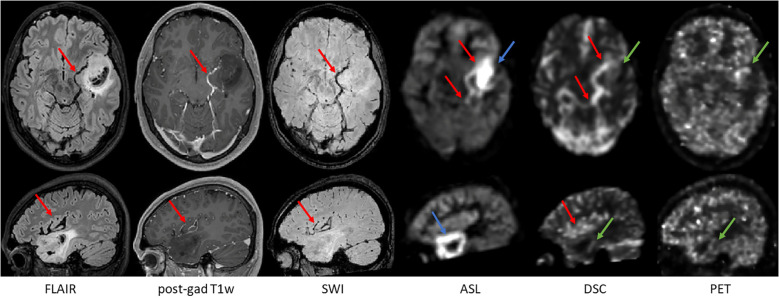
Participant 6 (grade 2 astrocytoma, IDH mutant, male, 20y) - a pair of representative axial (top row) and sagittal (bottom row) slices. On post-gadolinium T1w, the tumor shows inhomogeneous peripheral enhancement. On the axial images, a dilated draining vein is visible medial to the tumor on FLAIR, T1w, SWI, ASL, and DSC (red arrow). While the flow is likely high through this dilated vessel, the velocity is likely relatively low. This leads to a long arrival time, making the vessel visible on ASL and DSC (as indicated by the two red arrows), despite the absence of other macrovascular artifacts on ASL. On the sagittal images, a convolution of displaced and dilated M1 and M2 vessels running right above the tumor is visible on FLAIR, T1w, and SWI, with increased signal also on DSC, but not on ASL and PET (red arrow). Blood flow and volume comparable to that of normal GM were present in the tumor rim as assessed on PET and DSC (green arrows), and on the arterial blood volume (aBV) image of PET (not shown), respectively. ASL shows a very high signal in the entire tumor rim (over 300 mL/min/100g) (blue arrows). This CBF overestimation (normal GM CBF values are around 60mL/min/100g and lower than 100mL/min/100g) in ASL might be explained by the presence of numerous macroscopic vessels in the rim, in combination with delayed blood arrival. ASL, arterial spin labeling; CBF, cerebral blood flow; DSC, dynamic susceptibility contrast; GBM; glioblastoma; GM, gray matter; ROI, region of interest; WM, white matter.
